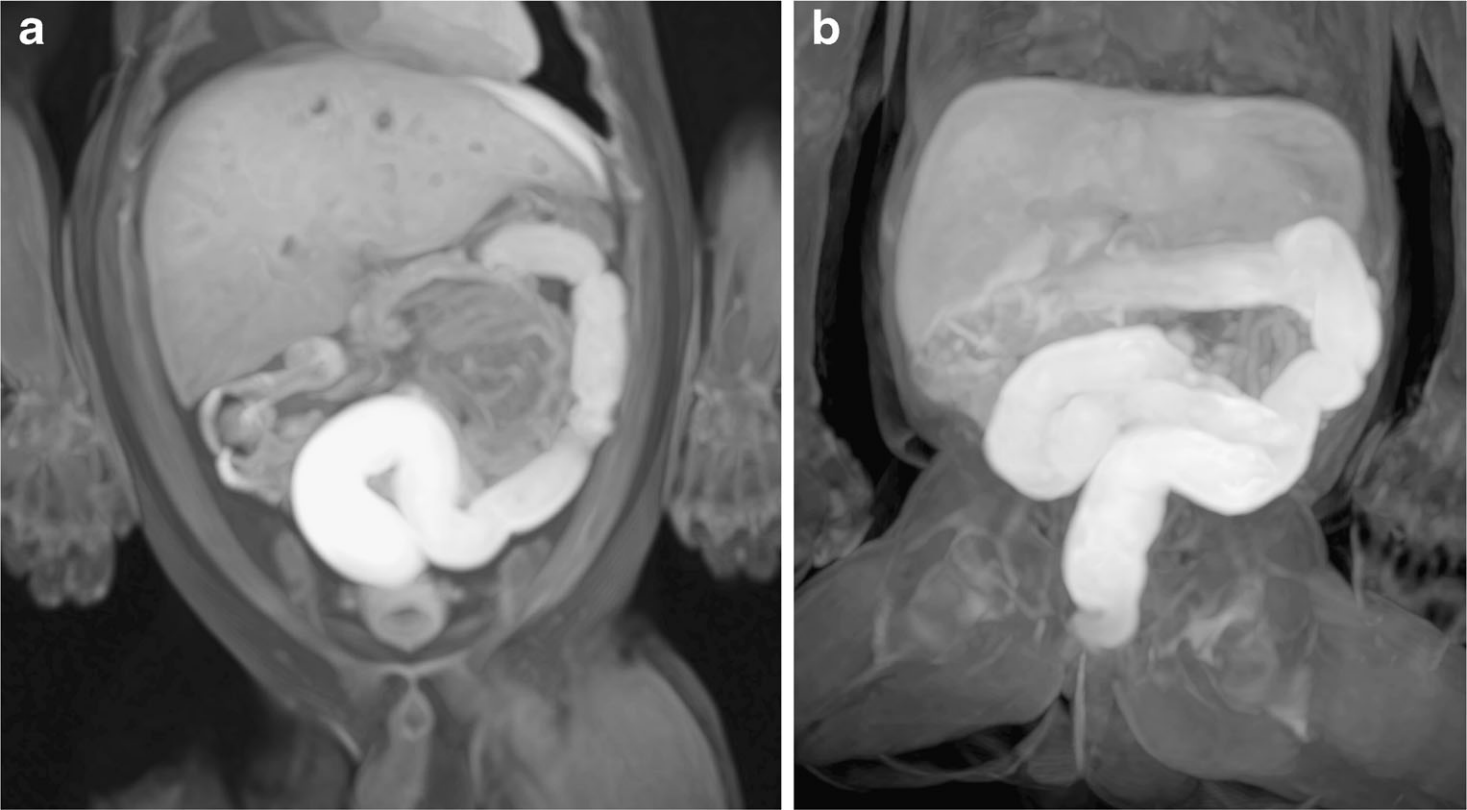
Normal meconium. Two examples of coronal T1-weighted VIBE MPR (volume interpolated breathhold examination multiplanar reformat) postmortem magnetic resonance imaging images from neonatal deaths, which show the normal gradually increasing high signal within the distal large bowel, consistent with normal meconium, similar to those seen on foetal or prenatal MRI. This can occur either predominantly from the sigmoid colon onwards (a) or from more proximal colon (b) The liver is of high signal on T1-W images (as opposed to low signal on T2-W images, e.g., Fig. 15)
